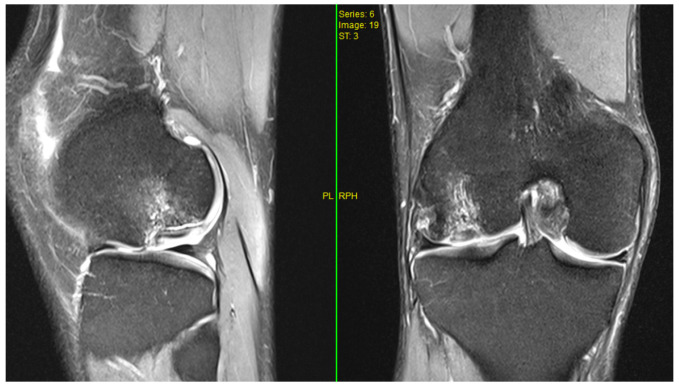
Preoperative MRI of the right knee with an osteochondral defect.
